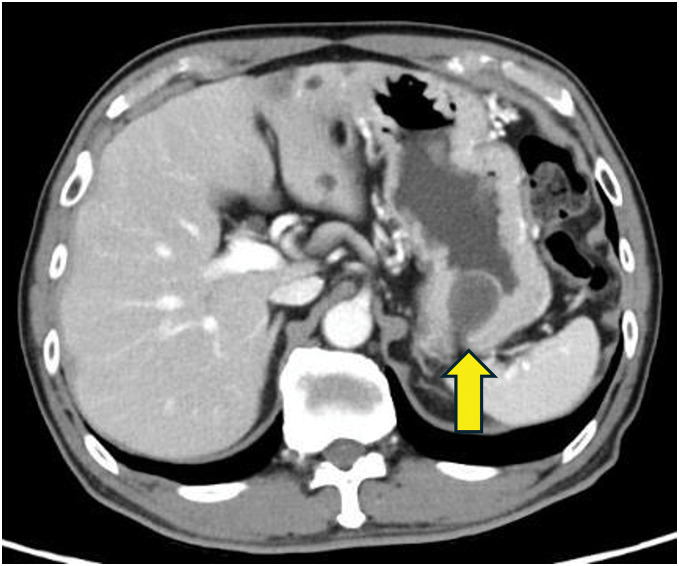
Contrast-enhanced computed tomography findings. Contrast-enhanced CT revealed a 28-mm ovoid submucosal tumor with mild heterogeneous enhancement in the posterior wall of the gastric body (arrow). No evidence of lymphadenopathy or distant metastasis was observed. The tumor was well-circumscribed and lacked invasive features, radiologically resembling a gastrointestinal stromal tumor (GIST).
